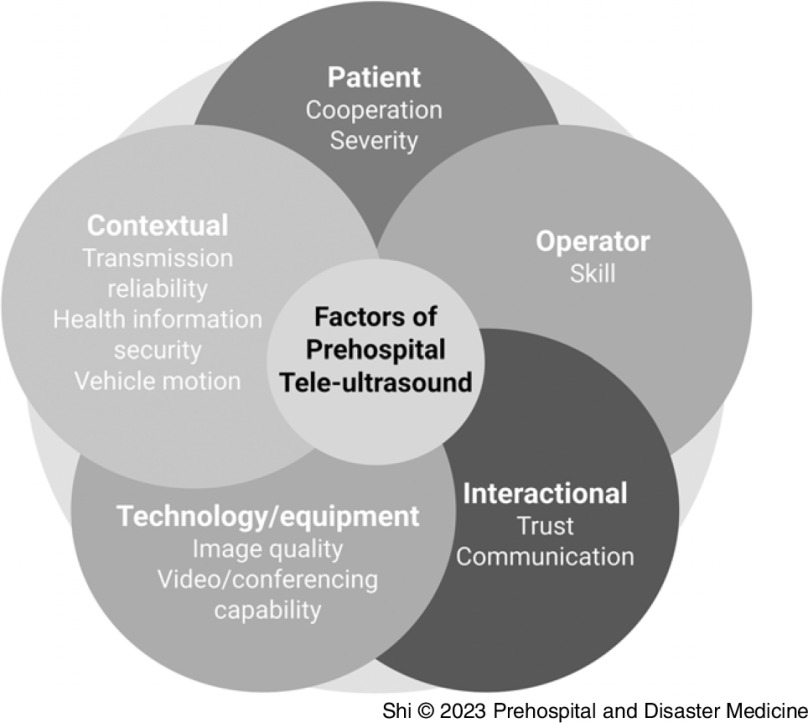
The 5 Pillars of Prehospital Tele-Ultrasound.
